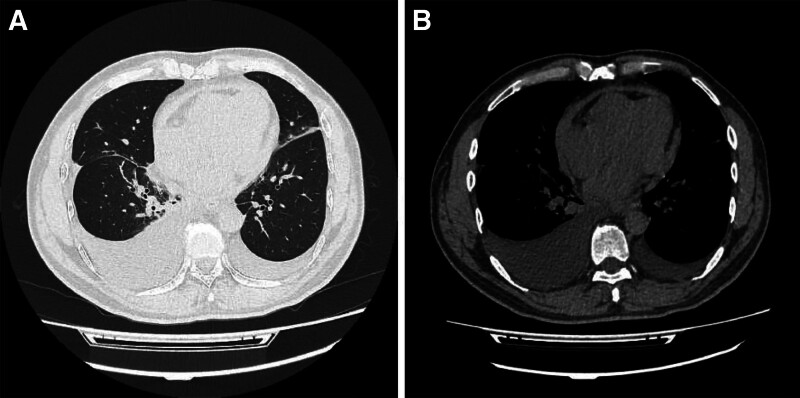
Pretreatment chest computed tomography (CT) imaging findings. (A) Axial lung window CT image shows bilateral pleural effusions, more prominent on the right side, with diffuse peribronchial thickening and patchy opacities in both lungs, indicative of inflammatory changes. (B) Axial mediastinal window CT image demonstrates significant pericardial thickening without evidence of pericardial calcification.
